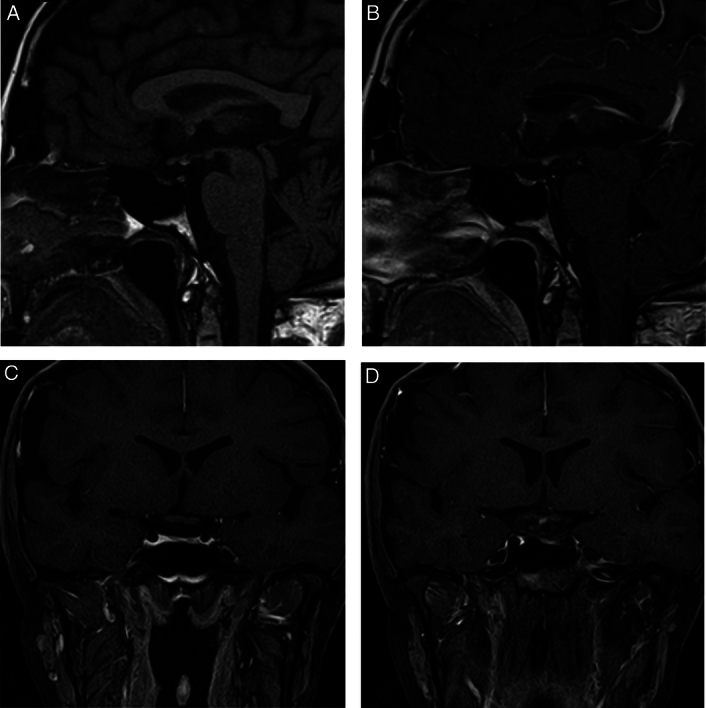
MRI findings: (A,B) Precontrast and postcontrast. T1-weighted sagittal images showing the presence and enhancing nodule at the level of the hypothalamus suggestive of an ectopic posterior pituitary with a hypoplastic anterior pituitary measuring only at 2.1 mm in height. (C,D) Precontrast and postcontrast fat-suppressed coronal images showcasing the absence of the pituitary stalk, and confirming the presence of the ectopic posterior pituitary.
